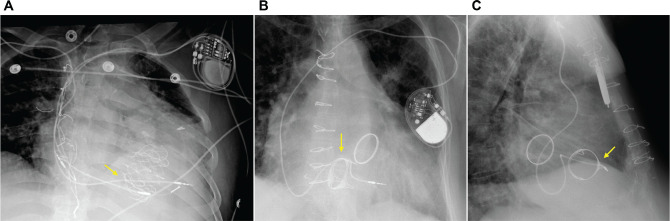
A: Frontal chest radiograph of a patient with two right ventricular leads that have been entrapped by a transcatheter tricuspid valve replacement (arrow). Posterior–anterior (B) and lateral (C) radiographs of a right ventricular lead that has been entrapped by a surgical tricuspid valve replacement (arrows).
